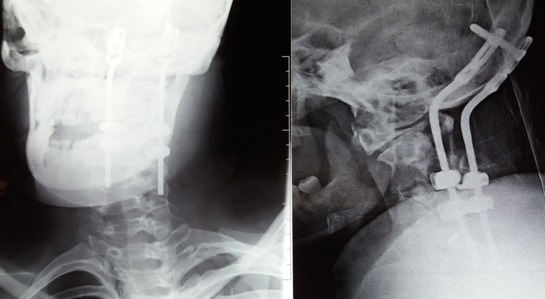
Post-operative X-Ray after occipito-cervical fixation
